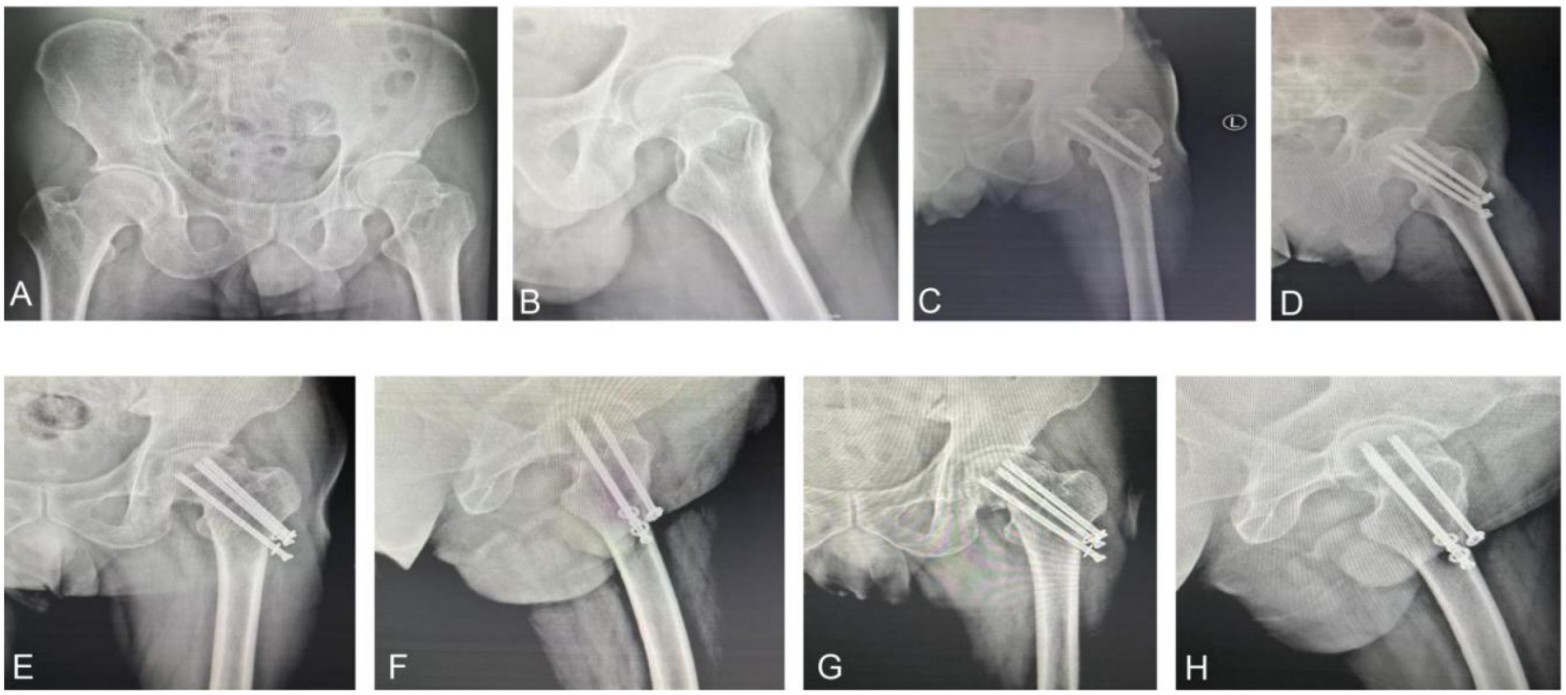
A 58-year-old case sustained a Pauwels type II FNF managed by CCS within 24 h subsequent to a fall. (A,B) Pre-op pelvic radiographs: AP (A) and lateral (B) views; (C,D) AP (C) and lateral (D) views on day 1 postoperatively; (E,F) AP (E) and lateral (F) at 3 months postoperatively, demonstrating loosening at the distal cannulated screw; (G,H) AP (G) and lateral (H) images at 6 months postoperatively, revealing complete loosening of all three screws with femoral neck shortening.
